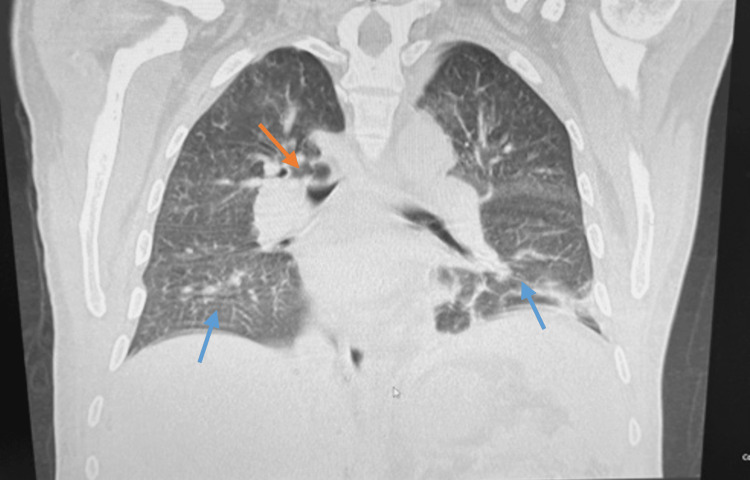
CT of the chest with contrast. CT of the chest (soft tissue) with contrast demonstrating an indeterminate roughly 7 mm nodule in the posterior right upper lobe (orange arrow). Large left and small right pleural effusions were also seen (blue arrows).
